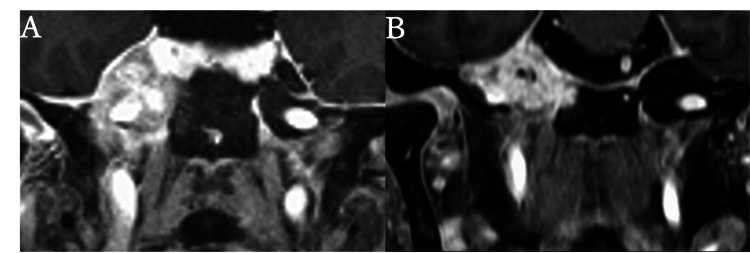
Contrast-enhanced MRI (coronal view) before and after treatment. (A) A contrast-enhancing tumor is observed occupying Meckel’s cavity. (B) The metastatic lesion in the right petrous bone shows an overall reduction in volume.
